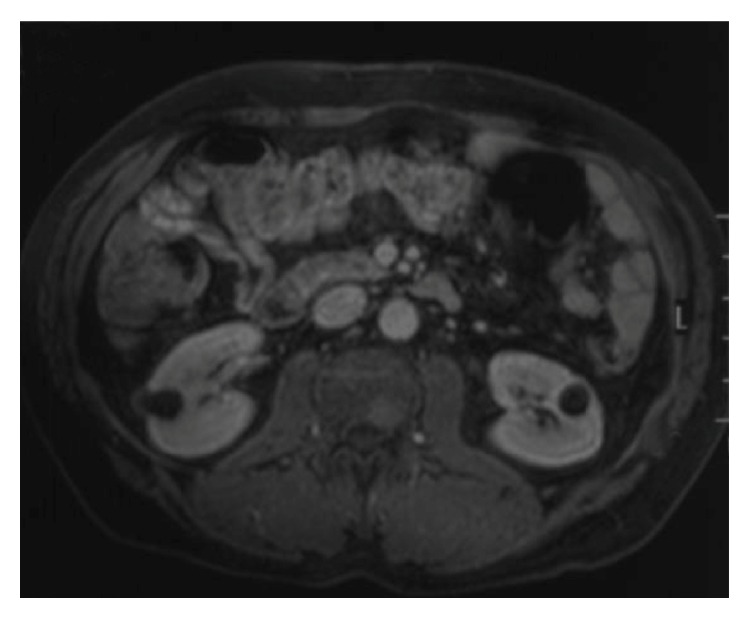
Abdominal MRI on T1-weighted axial imaging revealed a 1 cm wide heterogeneous metastatic lesion at the left side of posterior L2 and L3 vertebrae corpus.
